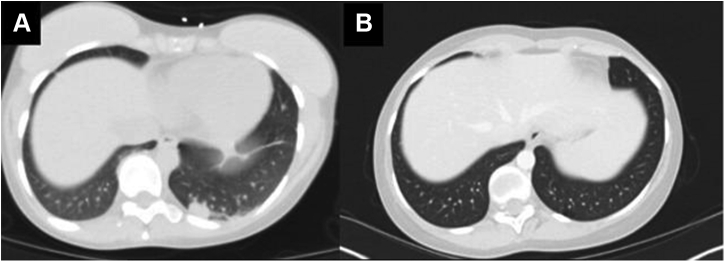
(*A*) CT chest with contrast at the time of diagnosis. (*B*) CT chest with contrast 4.5 years after diagnosis. CT, computed tomography.
